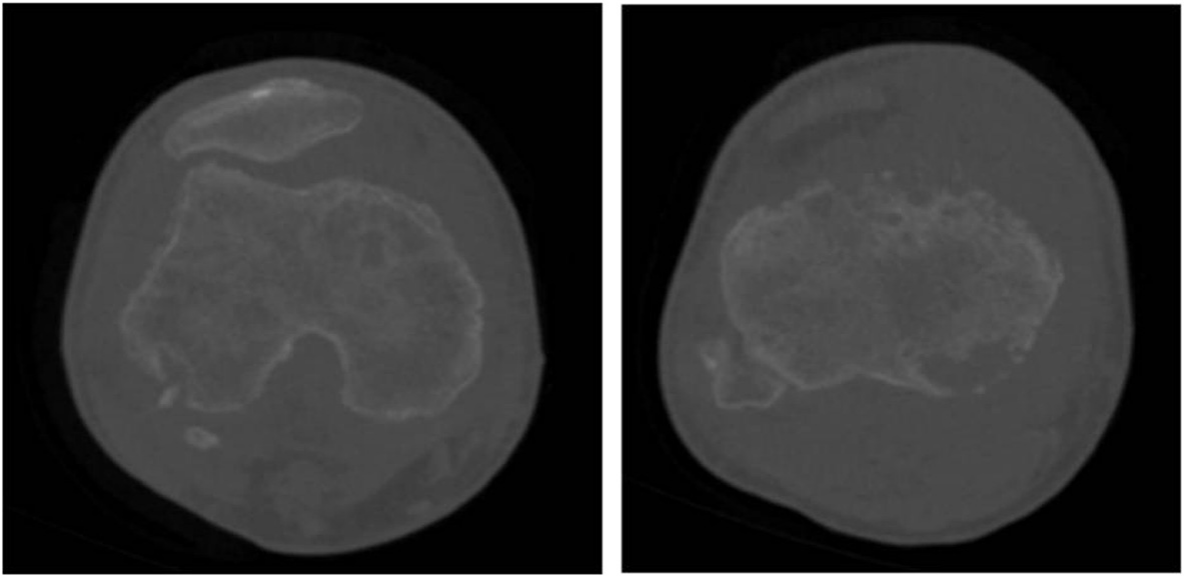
CT (axial) showing severe joint destruction and central and peripheral erosions
